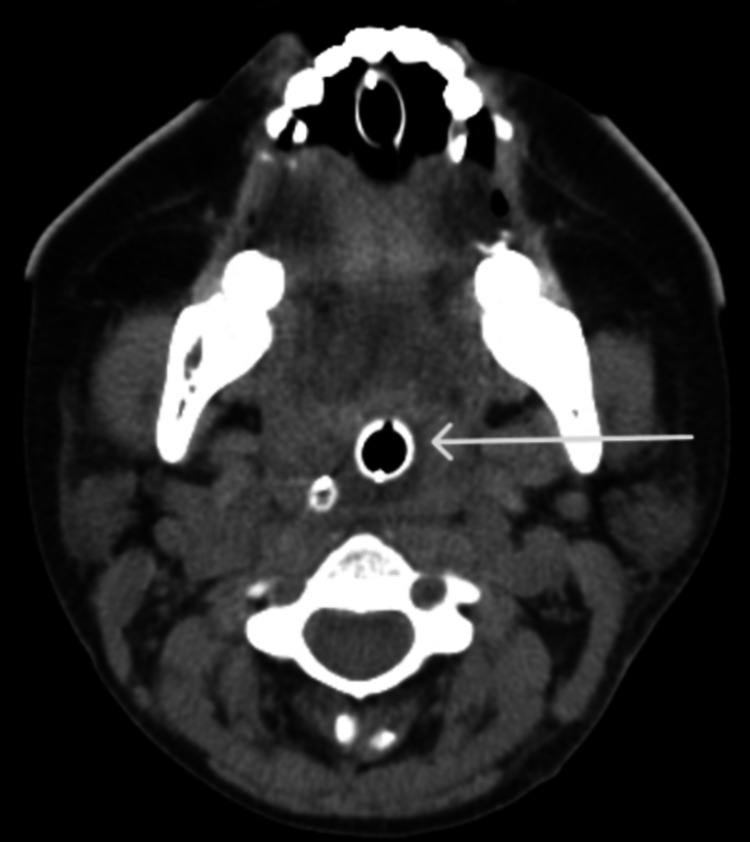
CT scan of the neck (axial section) The white arrow shows hypopharyngeal edema.
